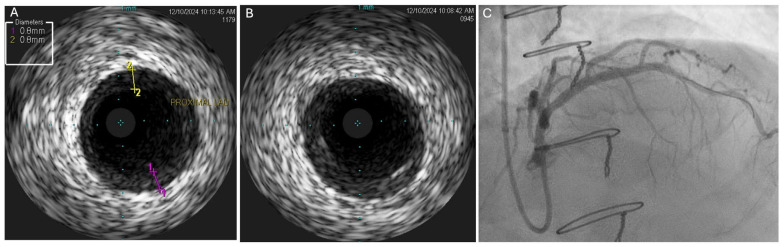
Discordance between findings of coronary angiography and IVUS. (A,B) IVUS of proximal LAD demonstrating mild concentric homogeneous intimal thickening in the proximal LAD with a maximal thickness of 0.8 mm, corresponding to severe intimal thickening (grade IV, as the thickening is greater than 0.5 mm in > 180 degrees of the vessel). (C) Coronary angiogram demonstrating very mild nonobstructive narrowing of the very proximal LAD which correlated with area of concentric homogenous thickening on IVUS. IVUS—intravascular ultrasound; LAD—left anterior descending artery.
